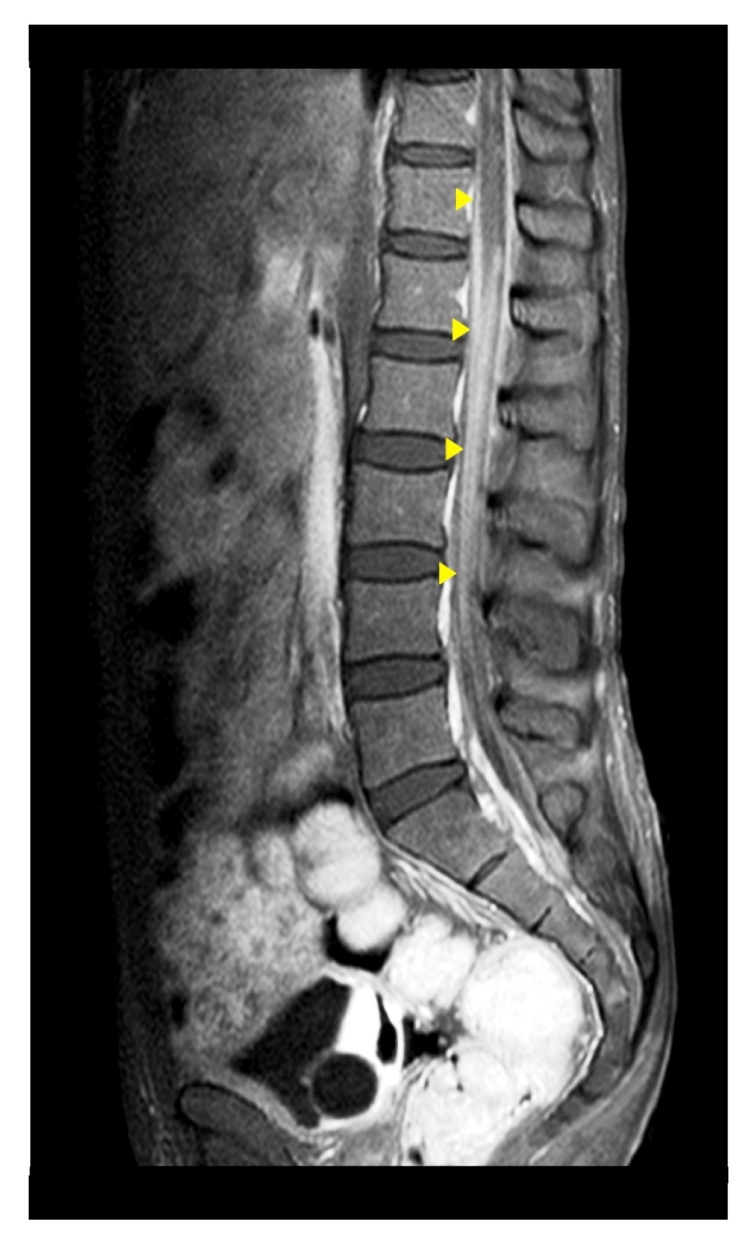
MRI revealing spinal meningeal enhancement surrounding the conus medullaris from the upper T12 level downwards (arrow) with an enhancement of the cauda equina nerve roots.
